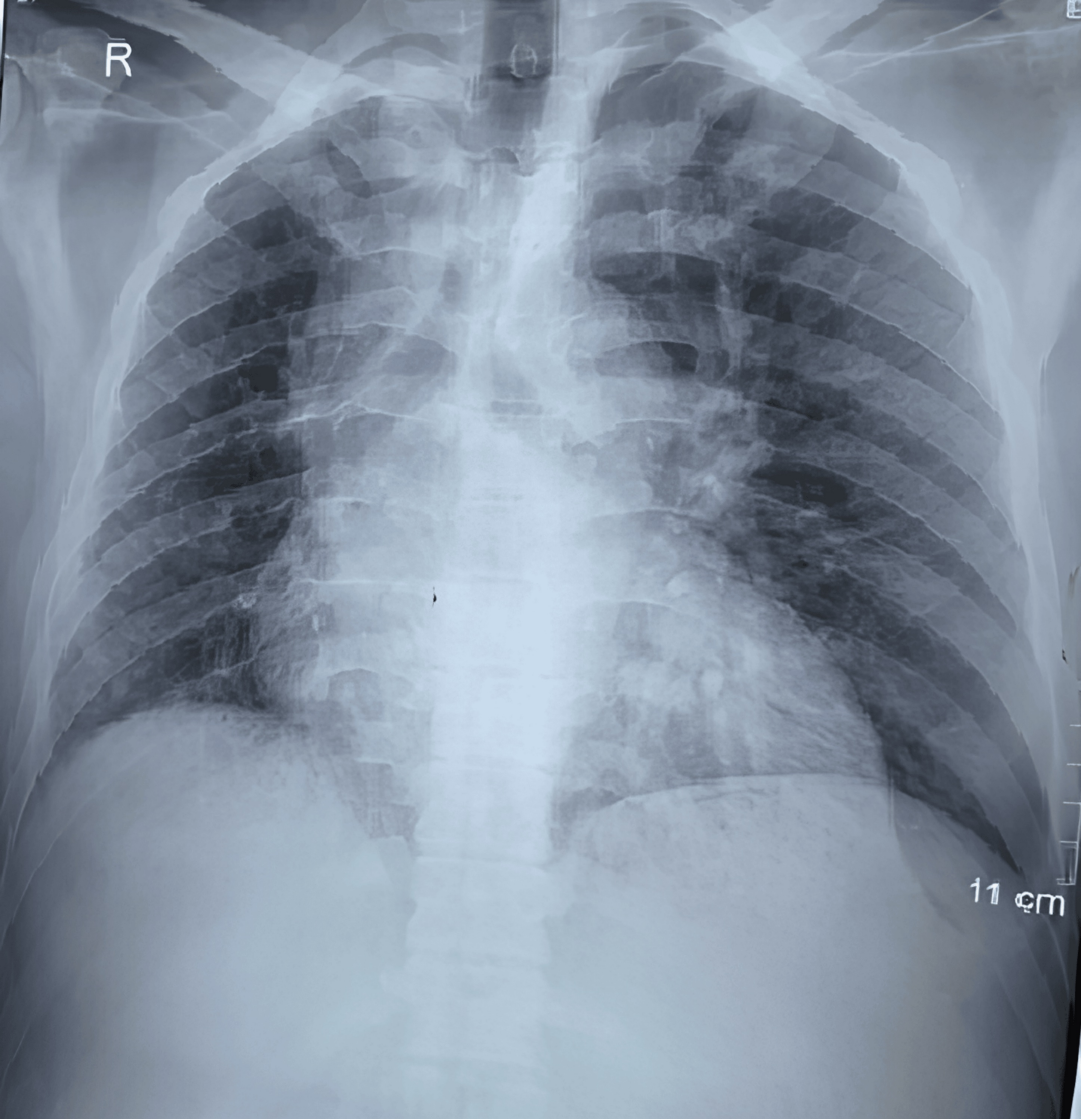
Front chest X-ray: retraction of the left pulmonary field, reduction of the left hilum, and hyperinflation of the right side
